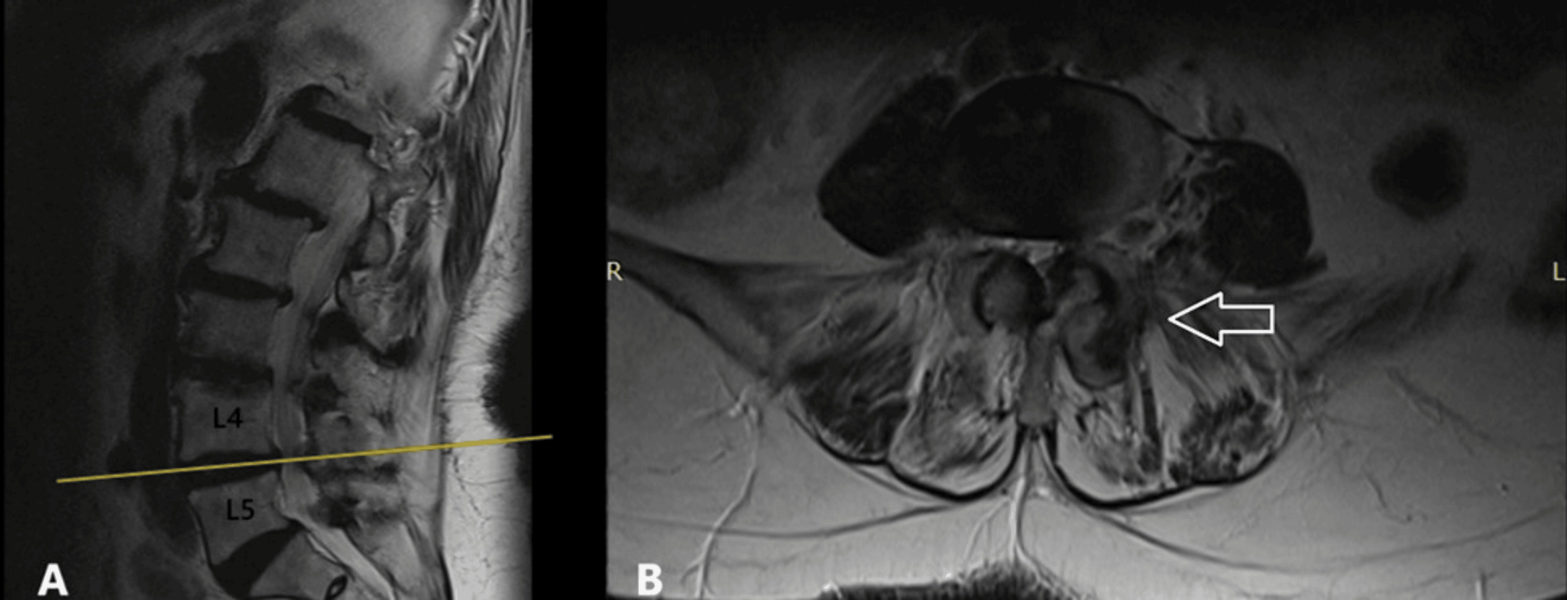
(A) T2-sagittal view and (B) T2-axial MRI views of the lumbar spine in fifth case. At the L4-L5 level, the white arrow indicates left-sided facet joint subluxation and facet hypertrophy.
